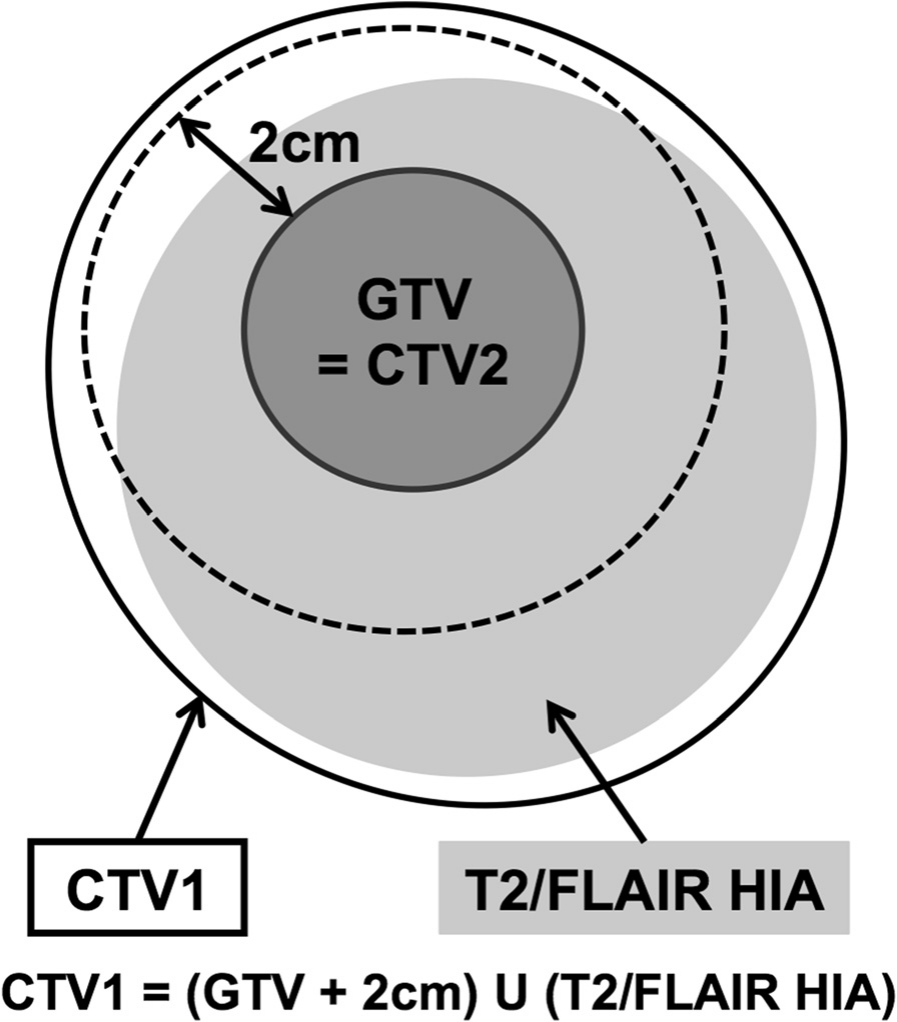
The target delineation method used in our hospital. GTV = gross target volume, CTV = clinical target volume, FLAIR = fluid-attenuated inversion recovery, HIA = high intensity area.
